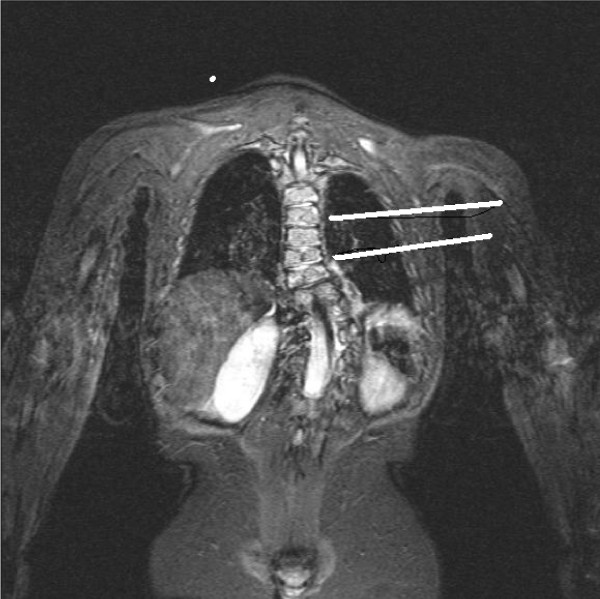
MRI T2-weighted Sequence, Coronal Plane Fat Saturation image. Complex Congenital Scoliosis Patient # 5. Superior line demonstrates concave zone growth plate deficiency and inferior line demonstrates a straight growth plate.
